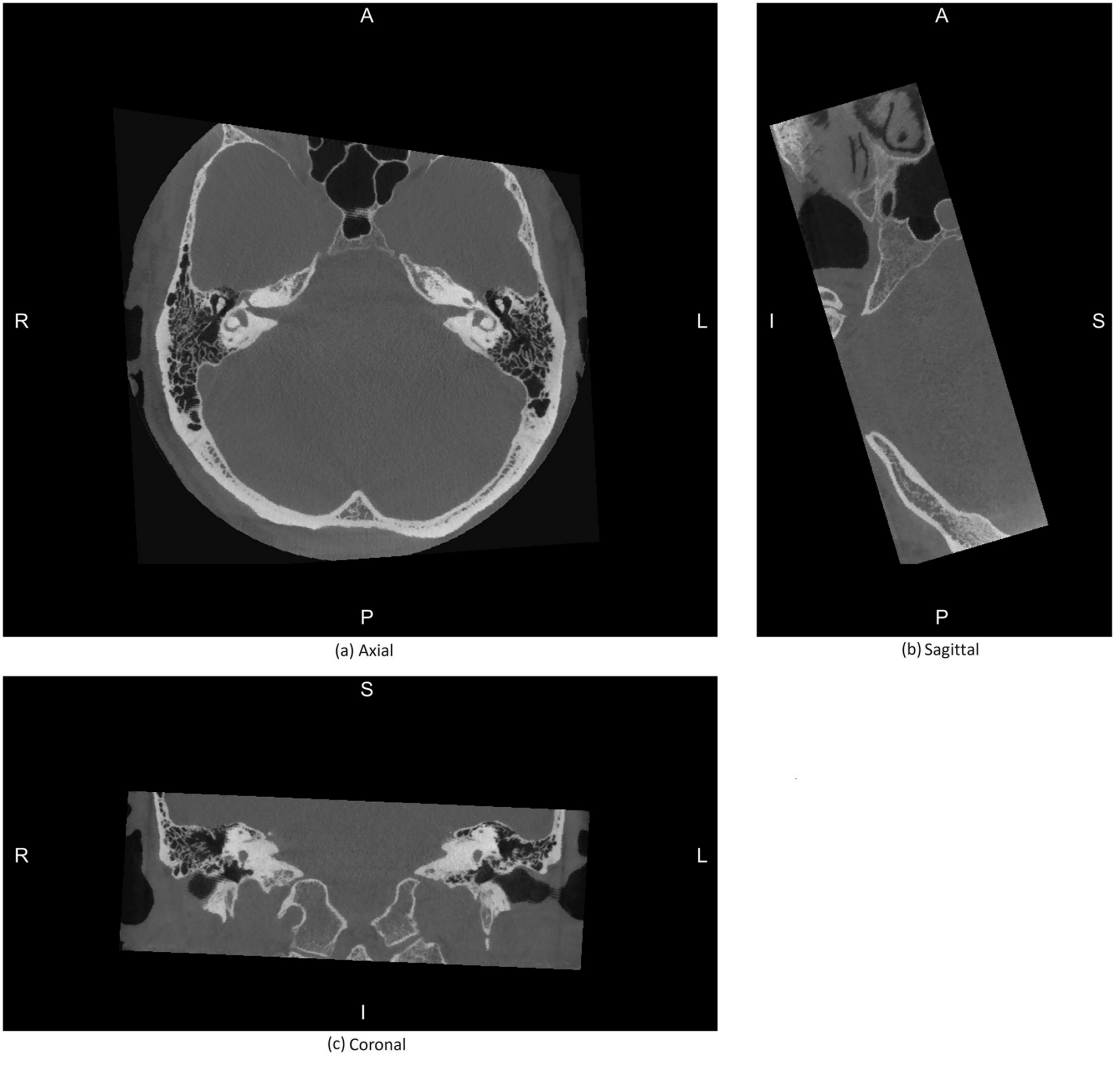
Mid slices of a CT image after it has been transformed to the proposed coordinate system. A, P, S, I, R, and L indicate anterior, posterior, superior, inferior, right, and left directions respectively.
